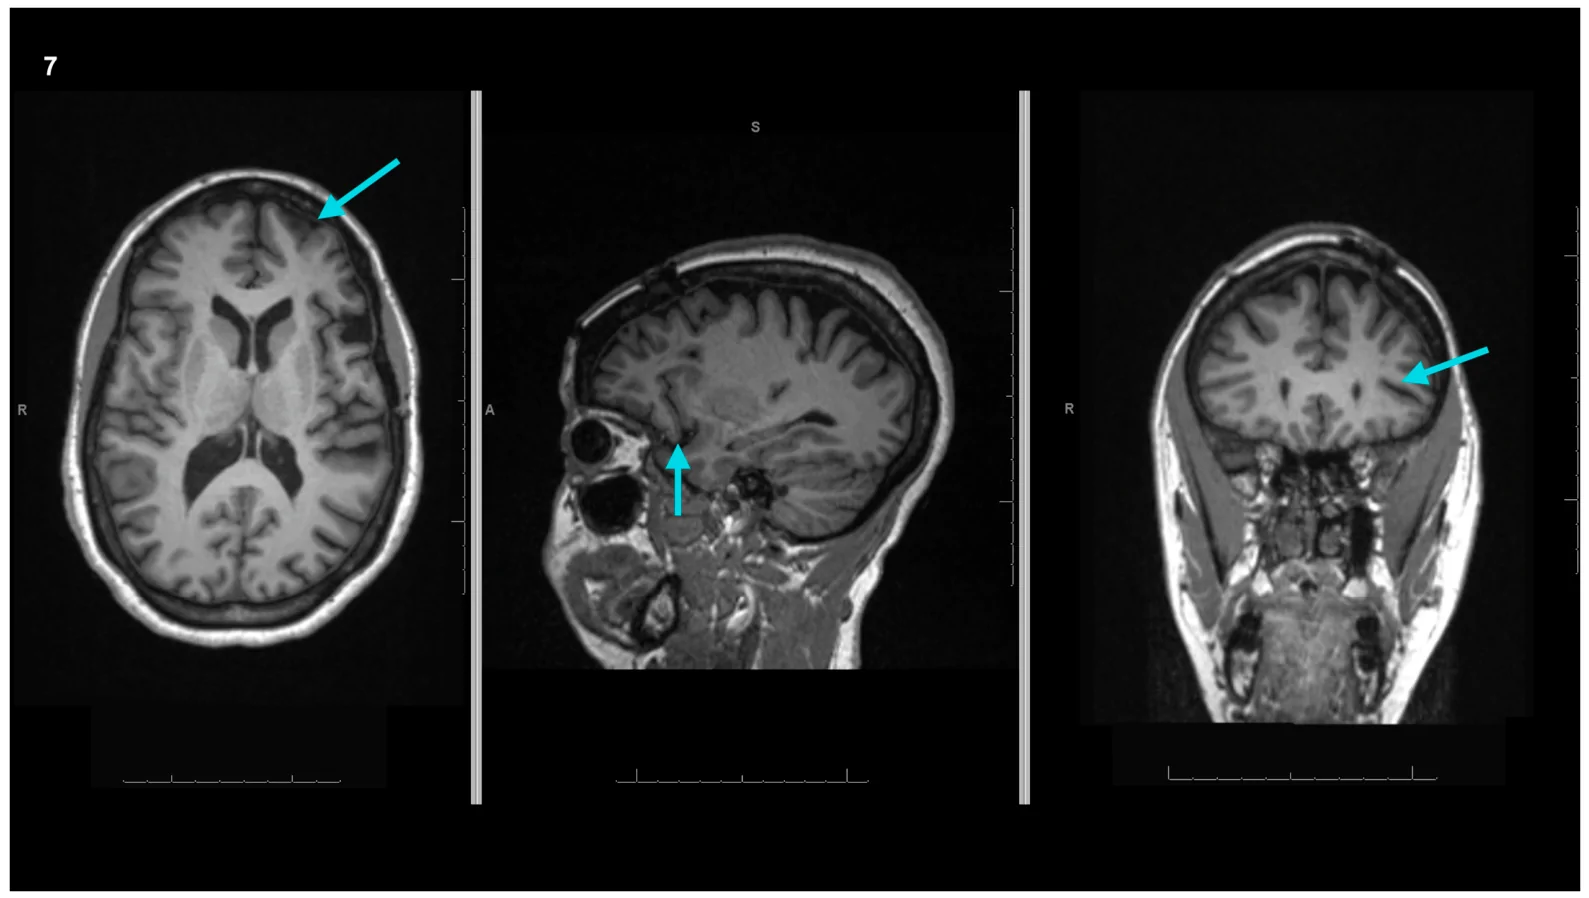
Case 2—Brain MRI re-review. After the MEG was presented in the patient management conference, the patient’s brain MRI was re-examined. The light blue arrow points to an abnormal gyrus and sulcus within the left orbitofrontal lobe (Magnetization-Prepared RApid Gradient Echo, MP-RAGE, sequences). These findings reinforce the importance of brain MRI revaluation after an MEG displays a cluster of ECDs within a specific brain region [60,61,63].
